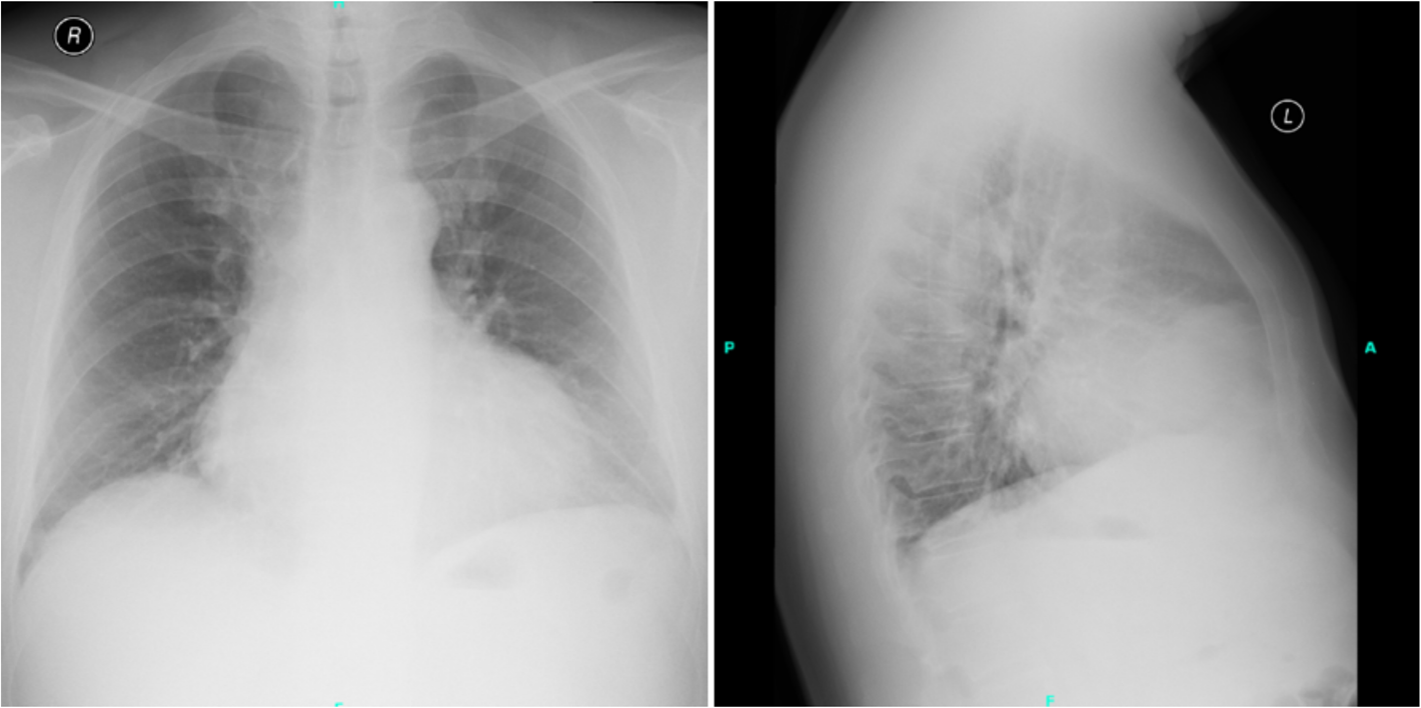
Chest X-ray image of the patient. Chest X-ray demonstrated small amount of pleural fluid in the left lateral sinus, 6.5 × 4.5 cm large consolidation or dystelectasis on the left side close to the significantly enlarged heart
